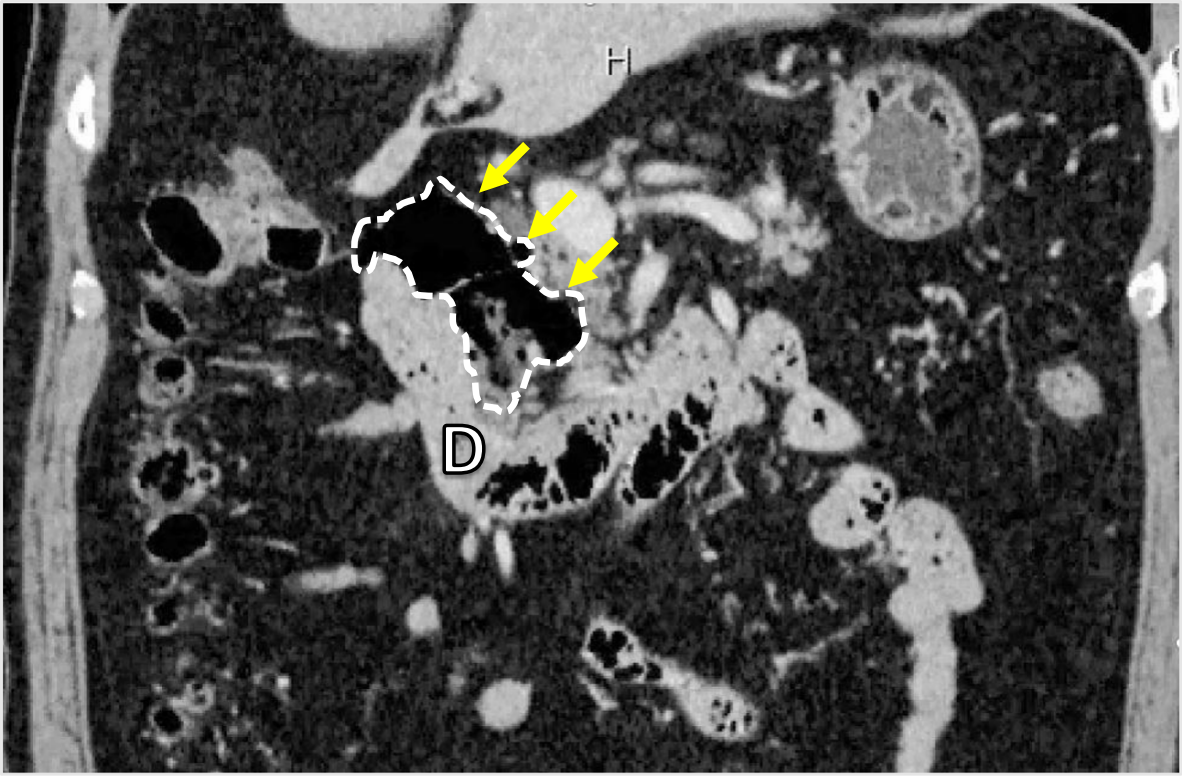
Computed tomography of case 1 before gastrectomy. A large periampullary diverticulum (yellow arrow) situated at the second part of the duodenum
